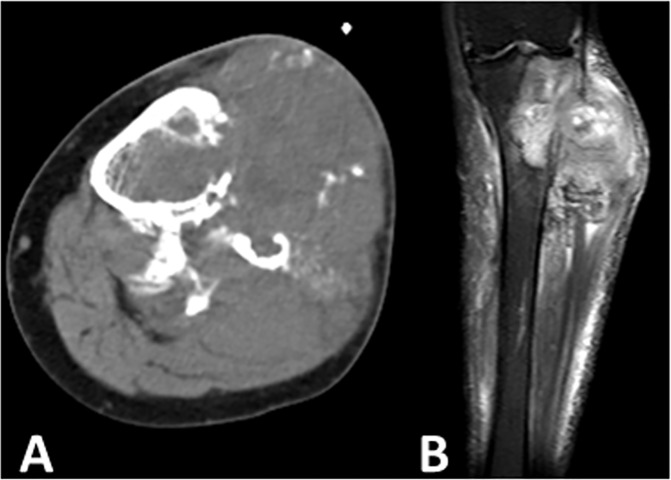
Dedifferentiated chondrosarcoma of the left proximal tibia and fibula in a 49-year-old woman (Case 9). (A).CT showed a flocculent shaped chondral matrix mineralization with marginal irregular shaped ossification and a soft tissue mass (B). Fat-suppressed coronal T2-weighted image showed heterogeneous signal intensity.
